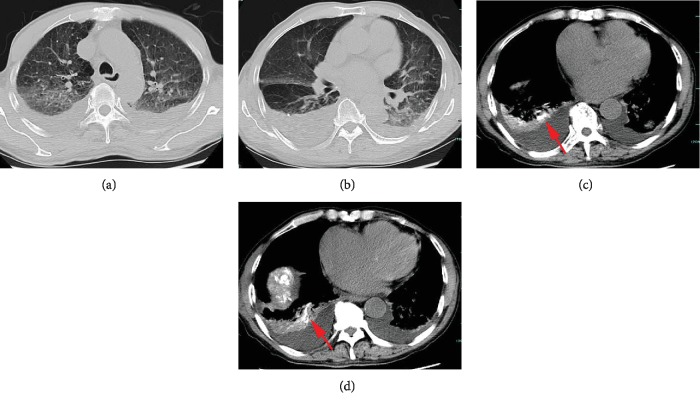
An 82-year-old man with HCC developed ARDS 24 h after the performance of TACE. The chest CT demonstrated diffuse pulmonary infiltration and bilateral pleural effusion (a, b). In addition, an obvious accumulation of Lipiodol (red arrows) was observed in the right lung lobe (c, d).
